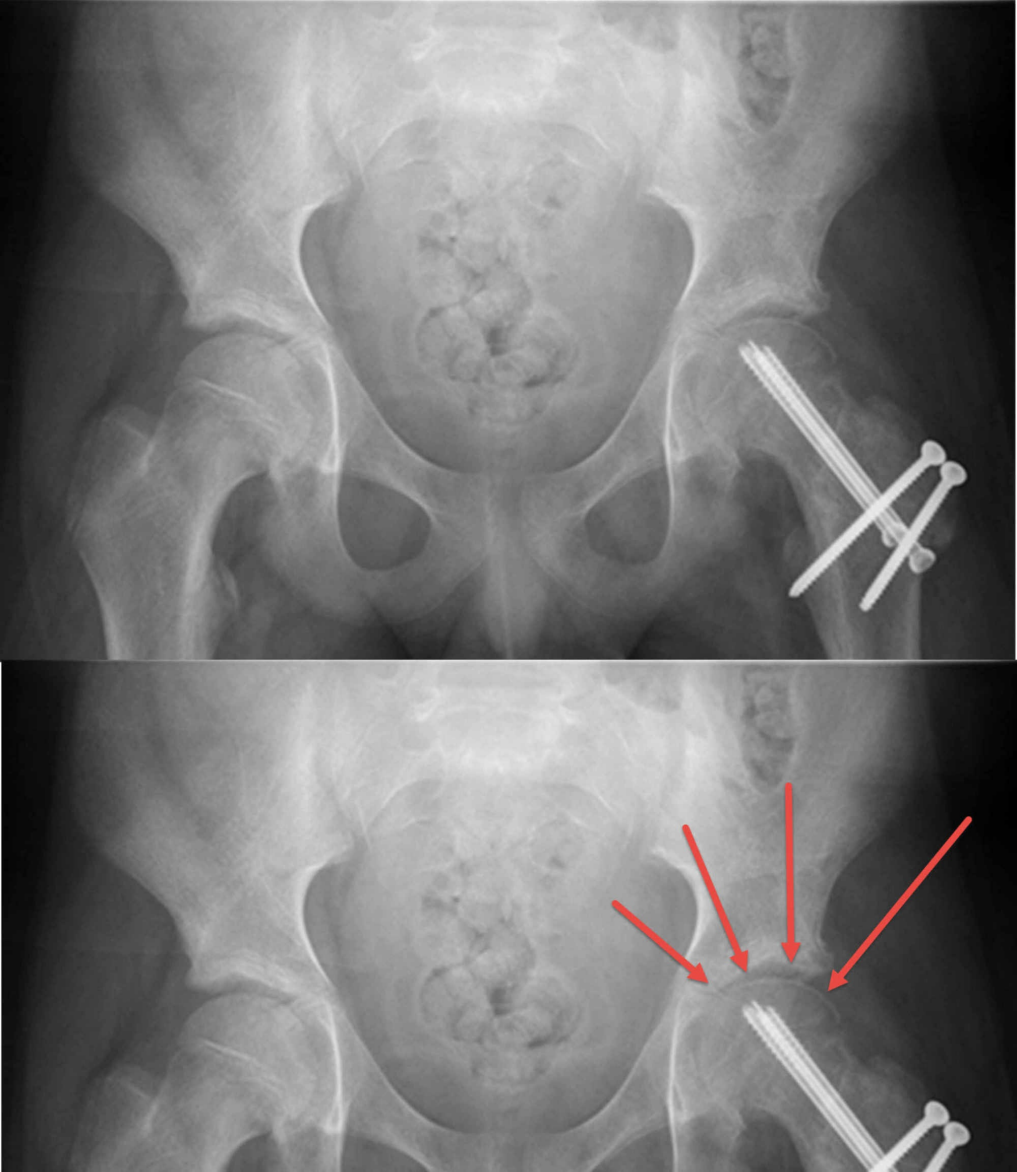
A pelvis x-ray moon or eid crescent sign Top image: postoperative plain pelvis x-ray (anteroposterior view) showing the anatomical reduction and the stabilizing screws. There is subchondral crescent-like osteopenia (moon crescent sign). Bottom image: close up image of the femoral head with the red arrows highlighting the subchondral crescent-like osteopenia (moon crescent sign).
